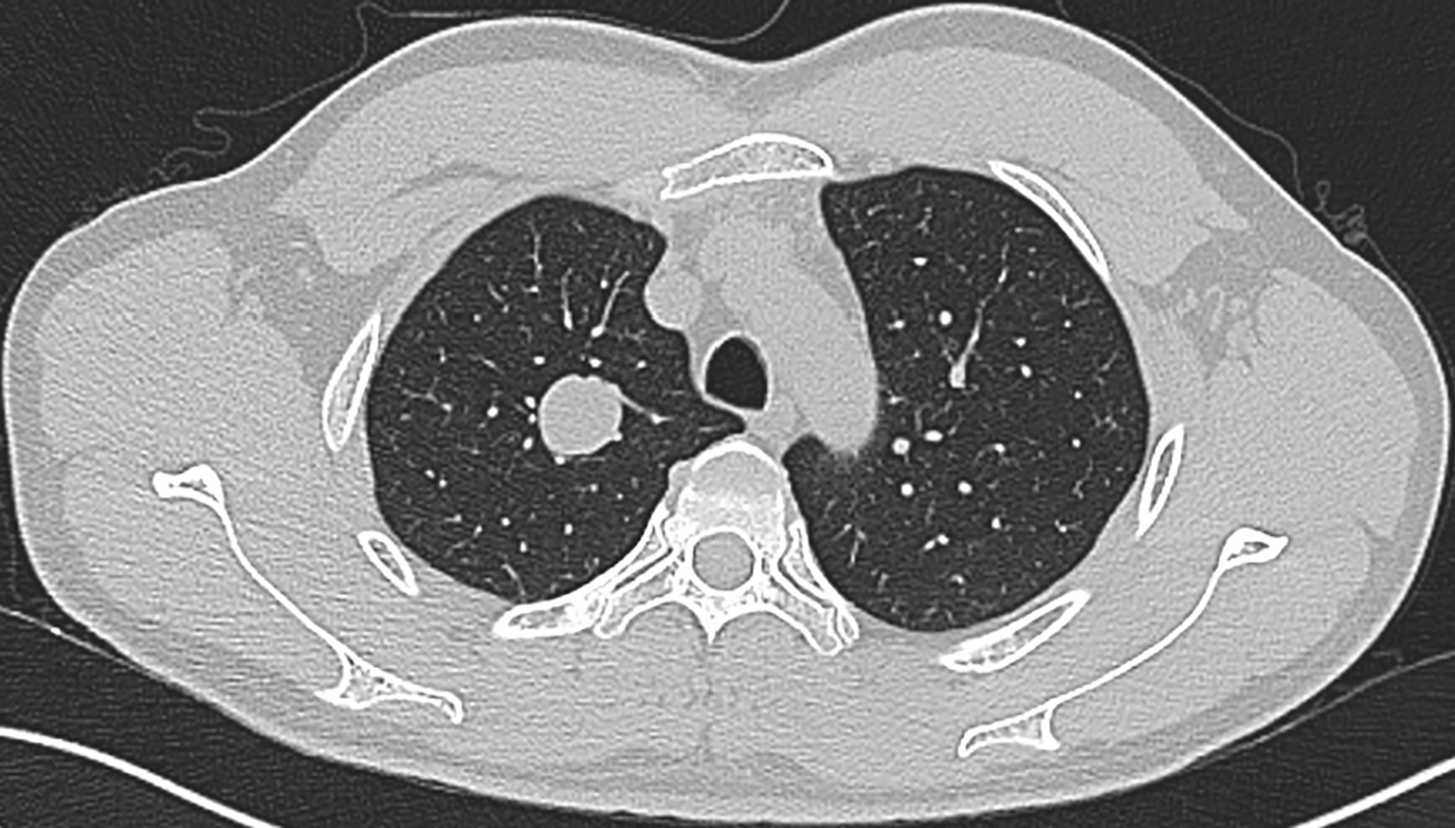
CT scanning of a case shows the lesion closely related to segmental bronchi.
